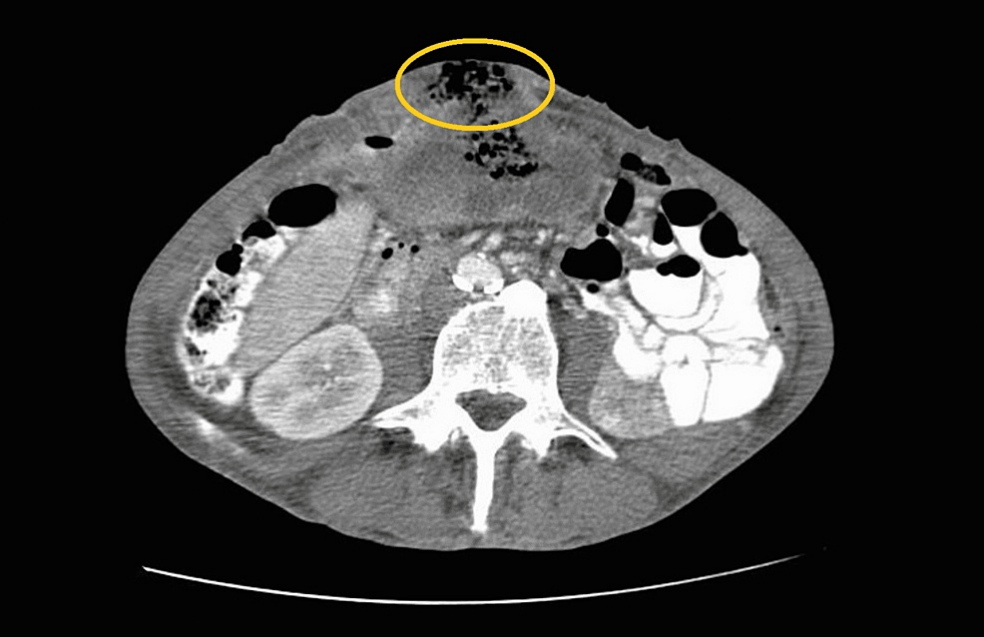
Coronal sections of abdominal CT images indicate a heterogeneous mass, presenting as enterocutaneous fistula (yellow circle).
